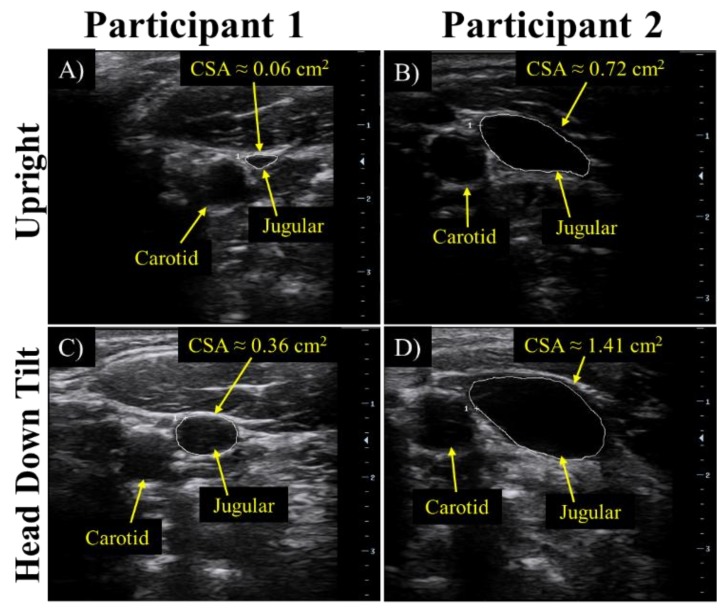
For the 70 s head down tilt at 50°, validation of the cephalad bio-fluid shift was determined by the cross-sectional area data obtained from ultrasound of the jugular vein as seen in upright position for (A) participant 1 and (B) participant 2, and for head down tilt for (C) participant 1 and (D) participant 2.
